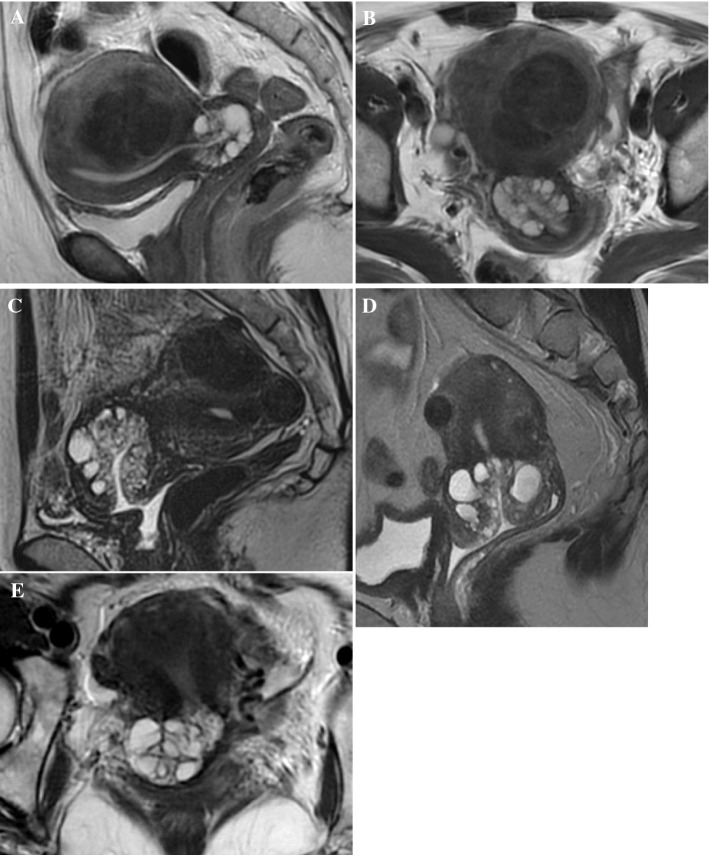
Typical MR Images. T2-weighed MR images of “cosmos” (A, B), and novel morphological categories of cystic components, “diffuse growth” (C, D) and “focal mass-like bulging” (E). A and B: A case of LEGH showing “cosmos”. There are multiple microcysts surrounded by macrocysts within the tumor at sagittal and axial T2-weighted image. C and D: Sagittal T2-WI showing “diffuse growth” observed in GAS cases. Microcysts exceeding 1/2 of the cervical stroma extend from external to internal cervical os. E: A case of atypical LEGH showing “focal mass-like bulging”. A cervical lesion is shown as an eccentric protrusion of round, mass-like multifocal cysts on axial T2-WI
